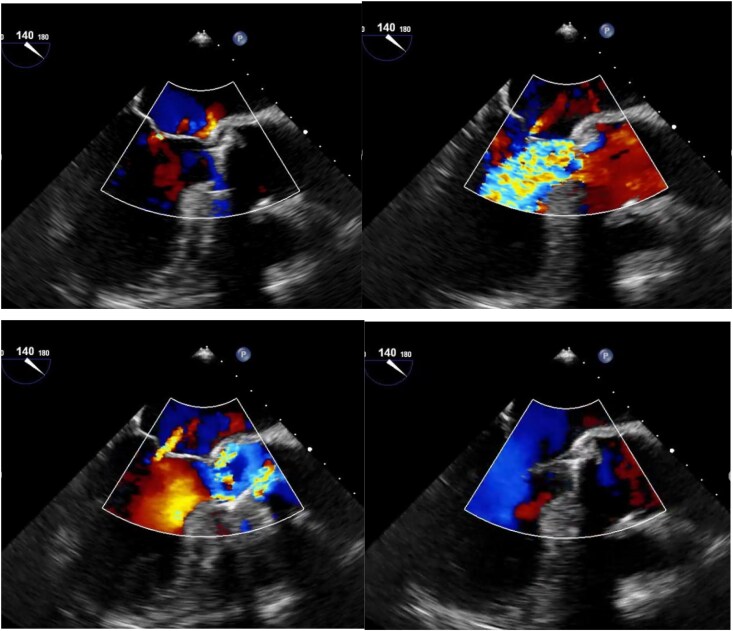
Transoesophageal echocardiogram mid-esophageal long-axis performed preoperatively.
